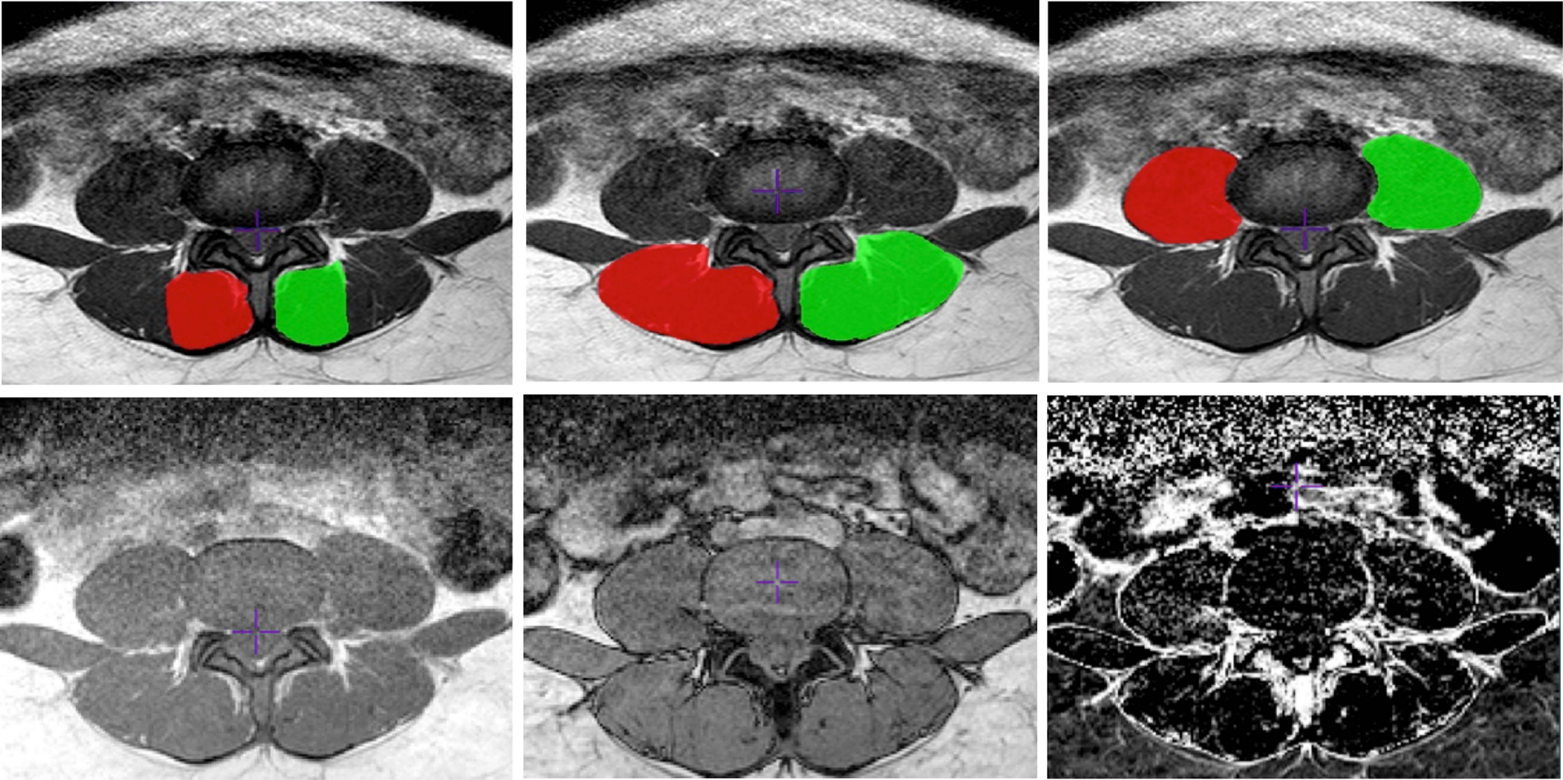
Superior line: Axial T1-weighted MRI at L4-L5 level with segmented lumbar muscles on Display® software. Right (green) and left (red) sides were indicated. *Left*: multifidus; *center*: erector spinae; *right*: psoas. Inferior line: Axial MRI sequences used to fat infiltration extraction. *Left*: In-Phase image; *center*: Out-Phase image; *right*: fat map.
